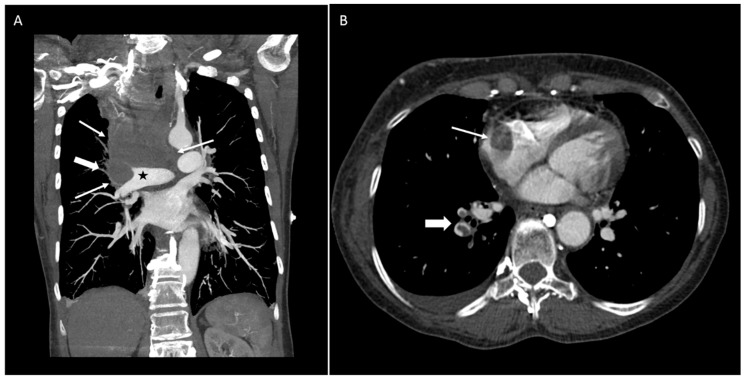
CT image of the thorax from outside the hospital showing (A) a large central mediastinal mass, occluding the pulmonary artery and extending into the right atrium, encasing the superior vena cava (SVC), abutting the trachea; and (B) pulmonary emboli at the right lower lobe.
